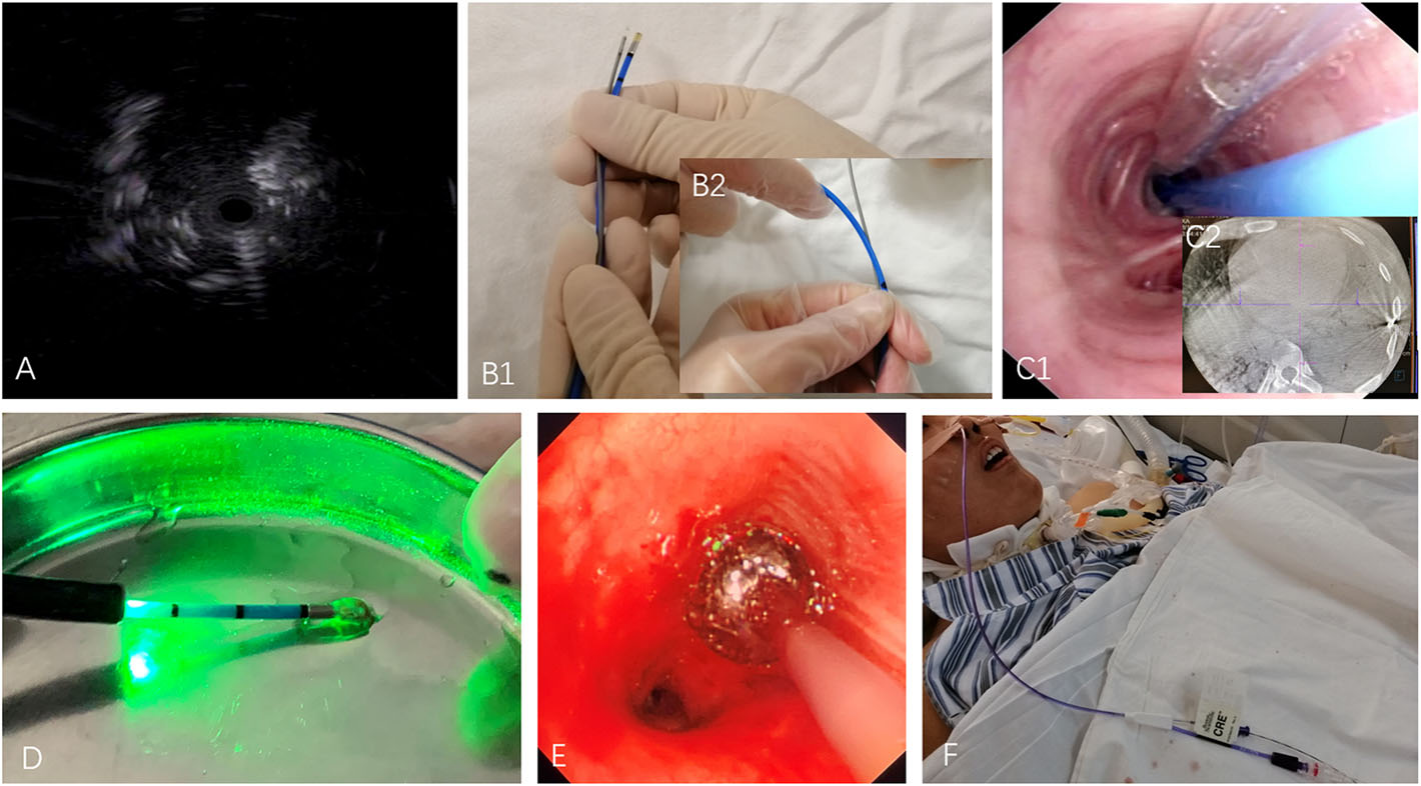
Radial probe endobronchial ultrasound (RP-EBUS) guided transbronchial lung cryobiopsy (TBLC) for acute respiratory distress syndrome (ARDS). a RP-EBUS screening of the target biopsy position. b Marking the biopsy distance on the cryoprobe compared to that on RP-EBUS. c1 Prophylactic placement of the bronchial blocker and insertion of the cryoprobe into the target segment. c2 Combined guidance with cone beam CT after placing the cryoprobe in patient 5. d Transbronchial lung cryobiopsy was performed after freezing for 4 s. e The bronchial blocker was filled to stop the bleeding. f Bronchial blockers were continuously placed in target bronchi for patients with massive bleeding
